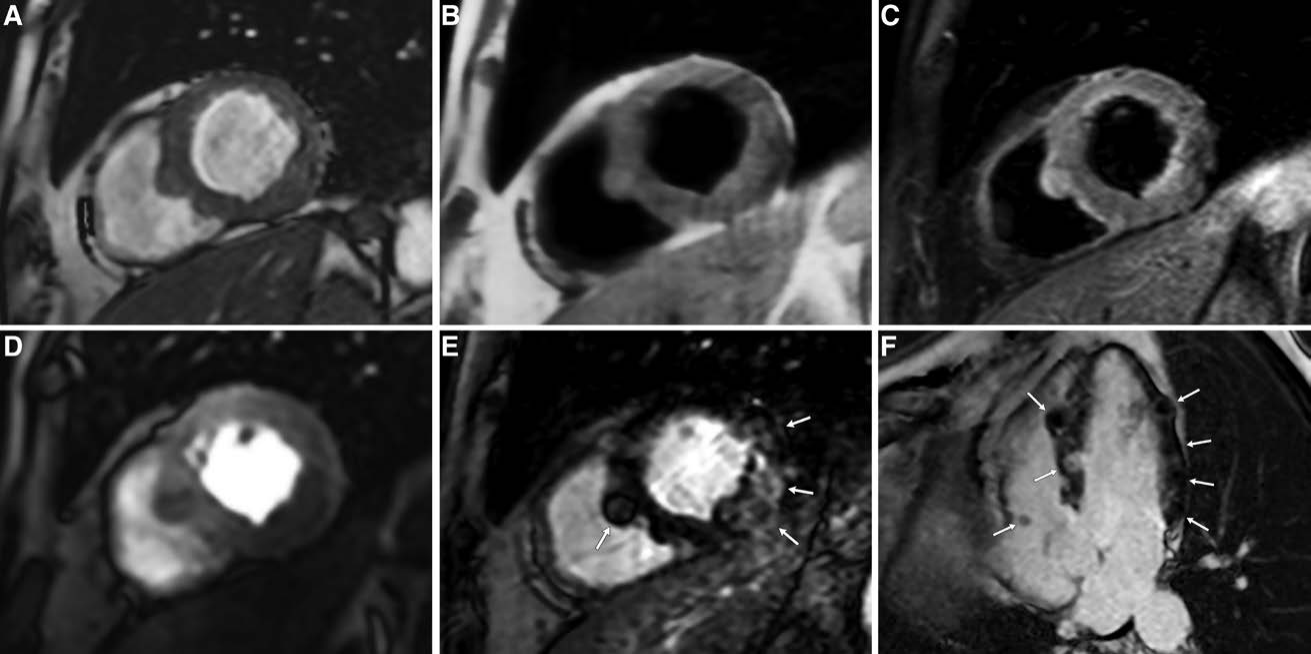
Cardiac magnetic resonance findings. Images were performed along the basal short axis (A–E) and horizontal long axis (F) views. Multiple nodular lesions are visible within the myocardial septal and inferior segments, mildly hypertense on cine-balanced steady state free precession (A), and T1-weighted (B) sequences and heterogeneously hyperintense to normal myocardium on T2-weighted short-tau inversion recovery sequence (C). First-pass perfusion imaging (D) shows reduced gadolinium enhancement compared with normal myocardium, mainly involving the inferior wall. Late gadolinium enhancement images (E and F) show heterogeneous enhancement of the nodular lesions (arrows), along the inferior wall of basal and mid segments, with rosary-beads-like appearance of the moderator band. A small nodular lesion is also visible in the inferior wall of the right atrium.
